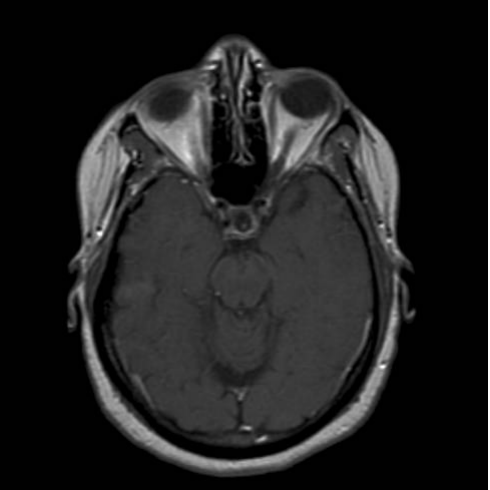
MRI brain with contrast showing near resolution of enhancement, stable on serial MRIs for 2 years.
